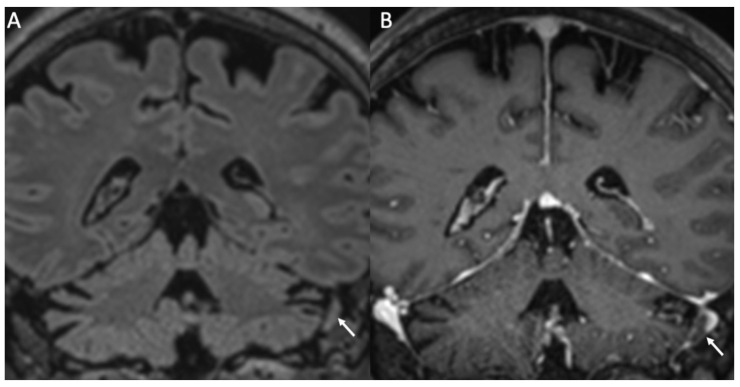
Coronal FLAIR (A) and enhanced T1 (B) show thrombosis of the right sigmoid sinus in a patient with Streptococcus pneumoniae meningitis (arrows in A,B).
